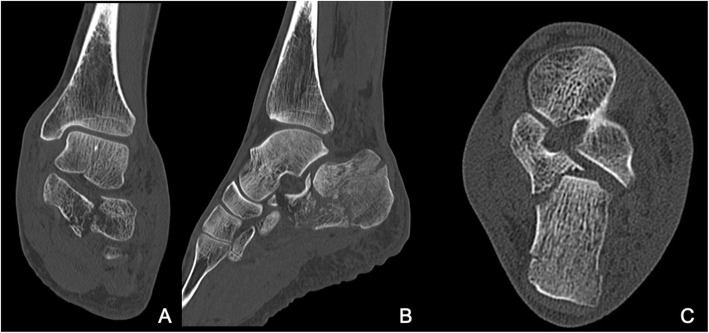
Case 1 Preoperative CT. Coronal (A), sagittal (B) and axial (C) views representing a Sanders IIb fracture type pattern
